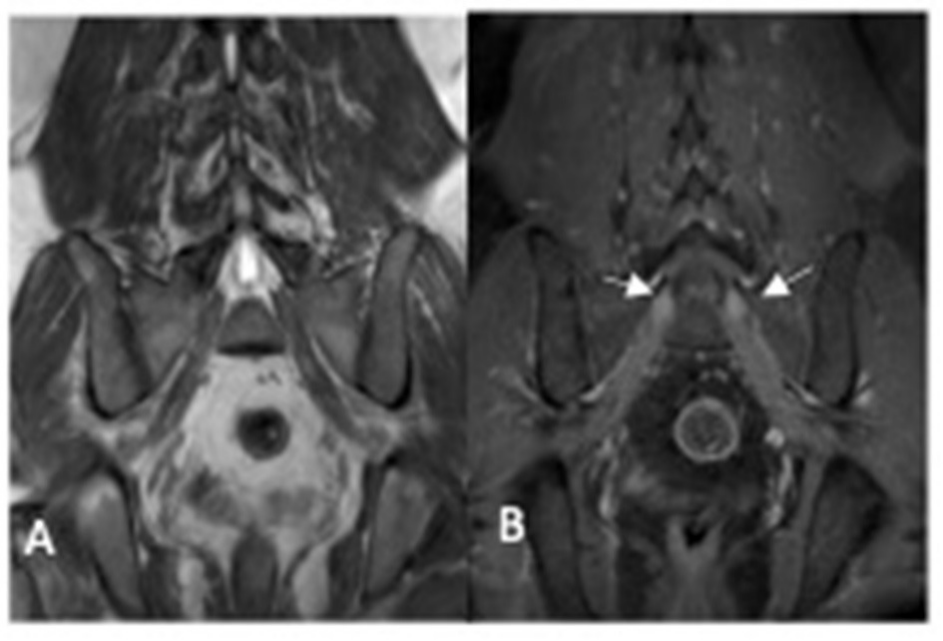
MRI lumbosacral plexus [patient two] showing enlarged sacral nerve roots with post-contrast enhancement (arrow). (A) coronol T1 non-contrast image; (B) coronal T1 post-contrast image.
